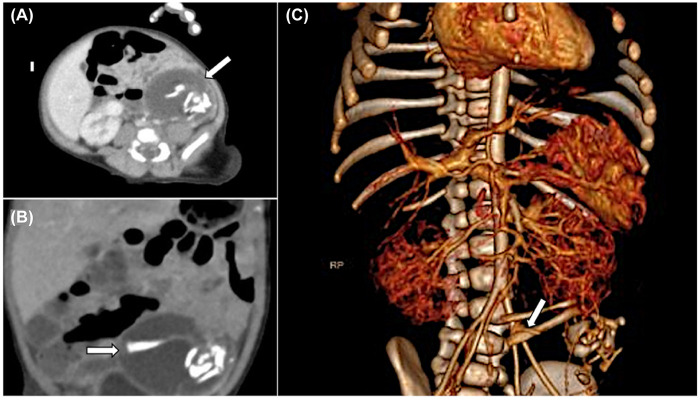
Abdominal CT images. (A) is a cross-section reveals a mixed-density space-occupying lesion in the lower left abdomen, marked by a white arrow. This lesion contains fat density shadows and patchy high-density bony structures. (B) is a coronal image. (C) is a three-dimensional reconstruction image of the tumor's pedicle is attached to the left iliac blood vessel.
